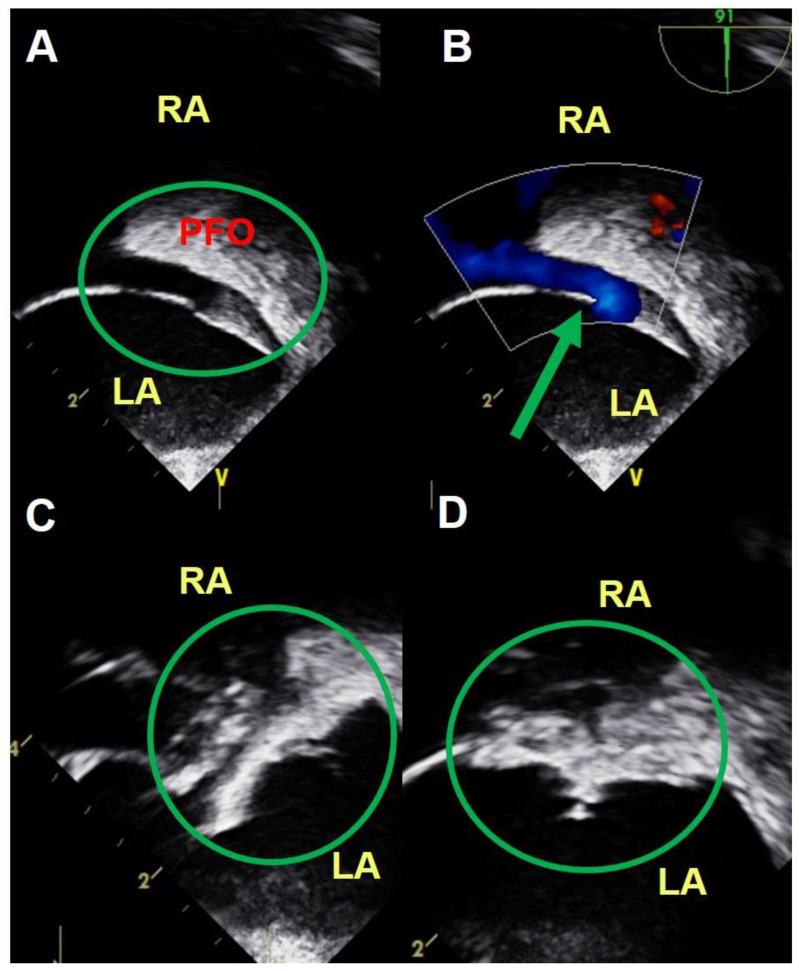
TEE-guided PFO device closure. A 40-year-old woman with a large PFO with resting left-to-right shunt underwent successful PFO device closure without any complication. TEE renders not only a two-dimensional PFO image (A) but also a good-quality color Doppler image of the resting left-to-right shunt via the PFO (B). An echocardiographer-cardiologist discusses with the operator about device deployment (C) and the result of the PFO device closure (D) during the procedure. TEE, transesophageal echocardiography; PFO, patent foramen ovale; RA, right atrium; LA, left atrium.
